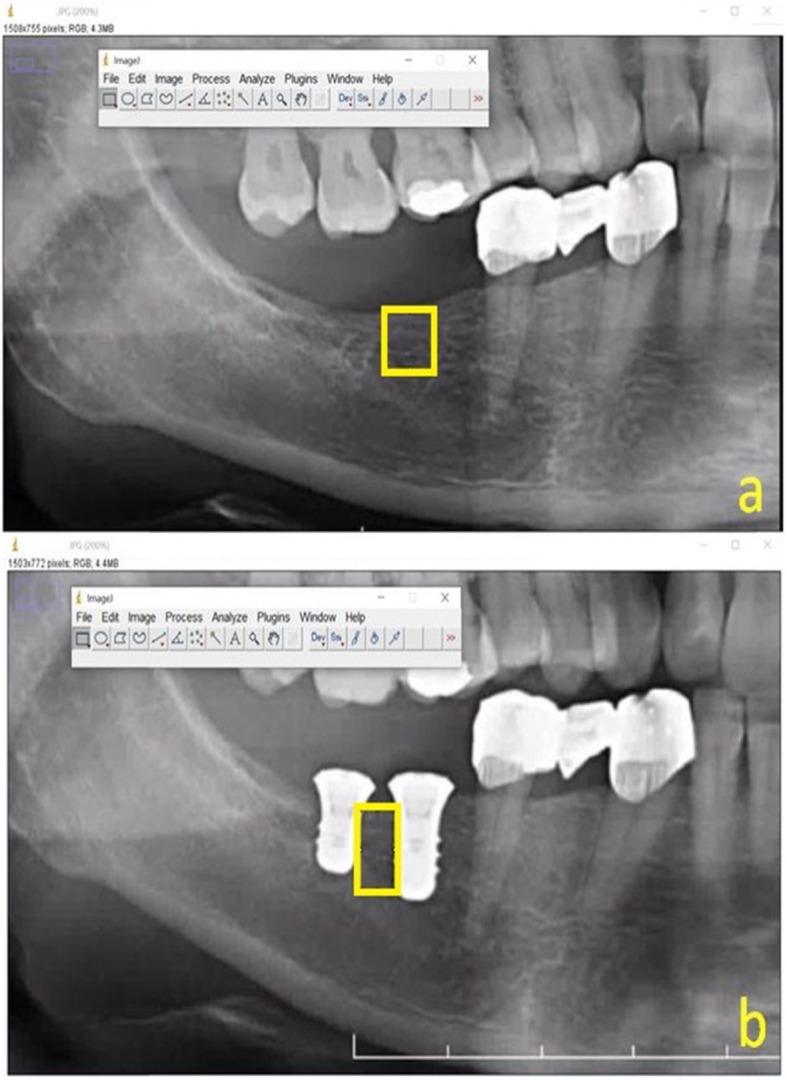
Region of interests (ROIs) were selected arbitrarily in a preoperative radiographic image and b a follow-up radiographic image
